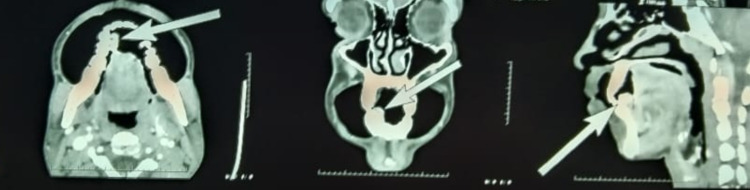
Contrast-enhanced CT (axial, coronal, and sagittal section) revealed a feeding artery on the gingiva on the lingual aspect in relation to 27, 28, 29 regions
